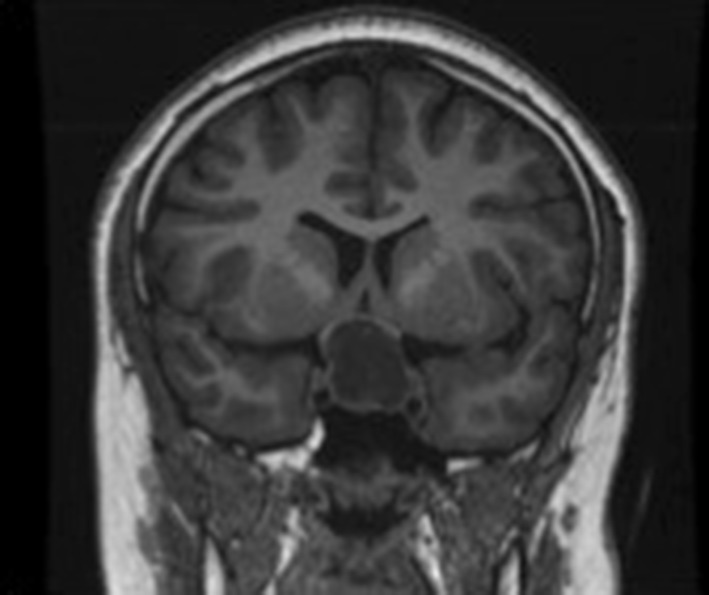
Child 4—Coronal unenhanced T1 MRI showing a tumour splaying the optic chiasm
